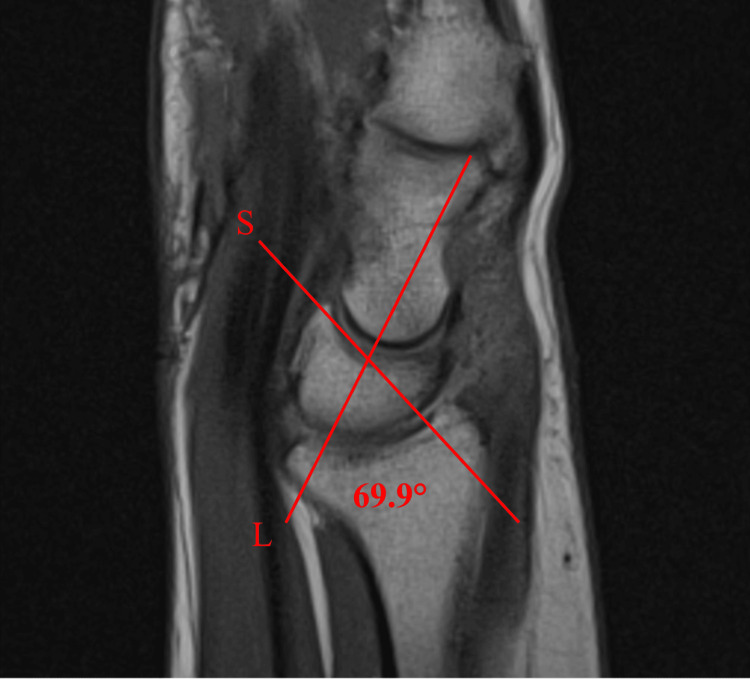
T1-weighted sagittal imaging shows a mild dorsal tilt of the lunate and mild DISI, which suggests scapholunate ligament injury/partial tear. The "S" line represents the axis of the scaphoid bone. The "L" line displays the axis of the lunate bone. Together, they create a scapholunate angle of 69.9 degrees. DISI = dorsal intercalated segment instability, S = scaphoid axis, L = lunate axis.
